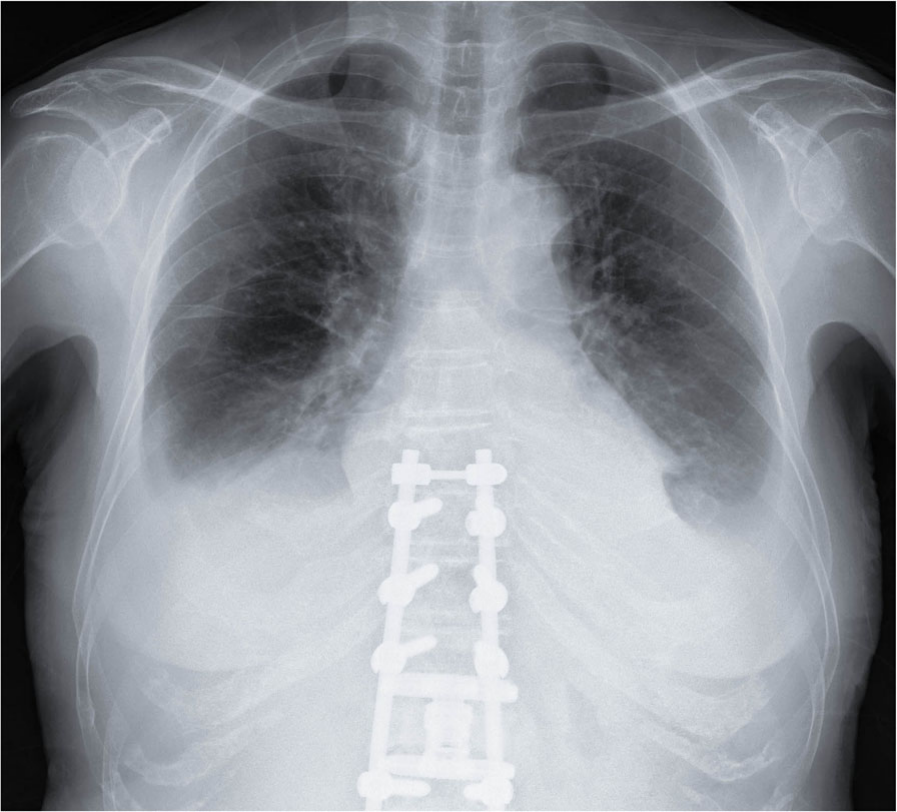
Bilateral pleural effusion was detected on a chest radiograph at the time of hospitalization
